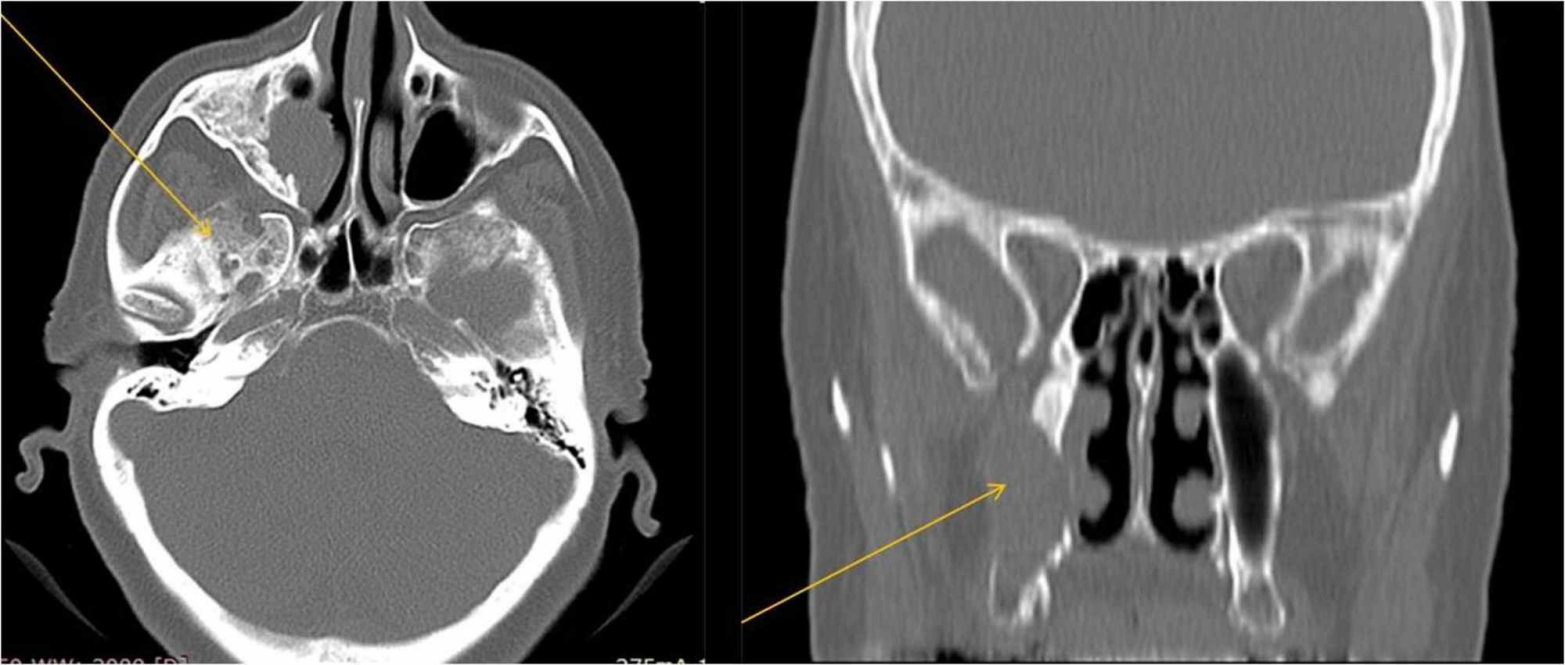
CT imaging of the paranasal sinuses, revealing a soft tissue lesion occupying the maxillary sinus and nasal cavity of the right side with disintegration of the outside and inside wall
